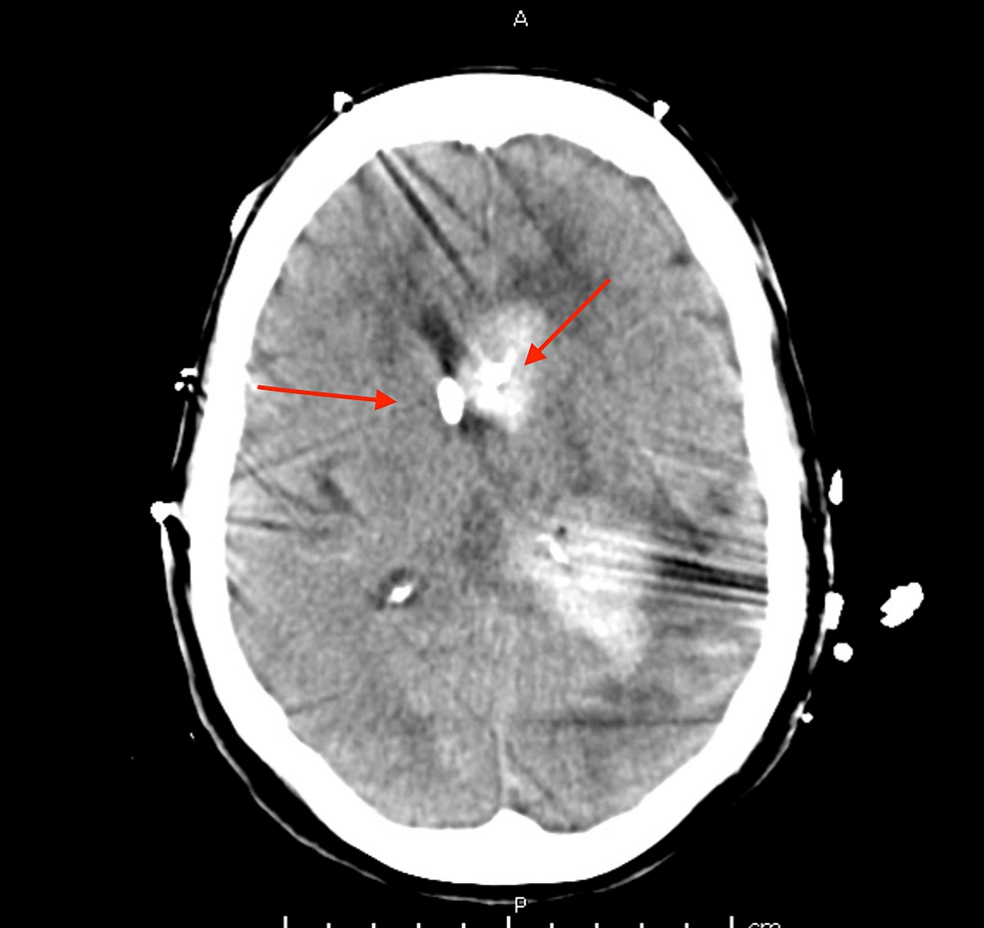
Head CT following placement of left-sided IRRAflow catheter Arrows indicate catheter tips
